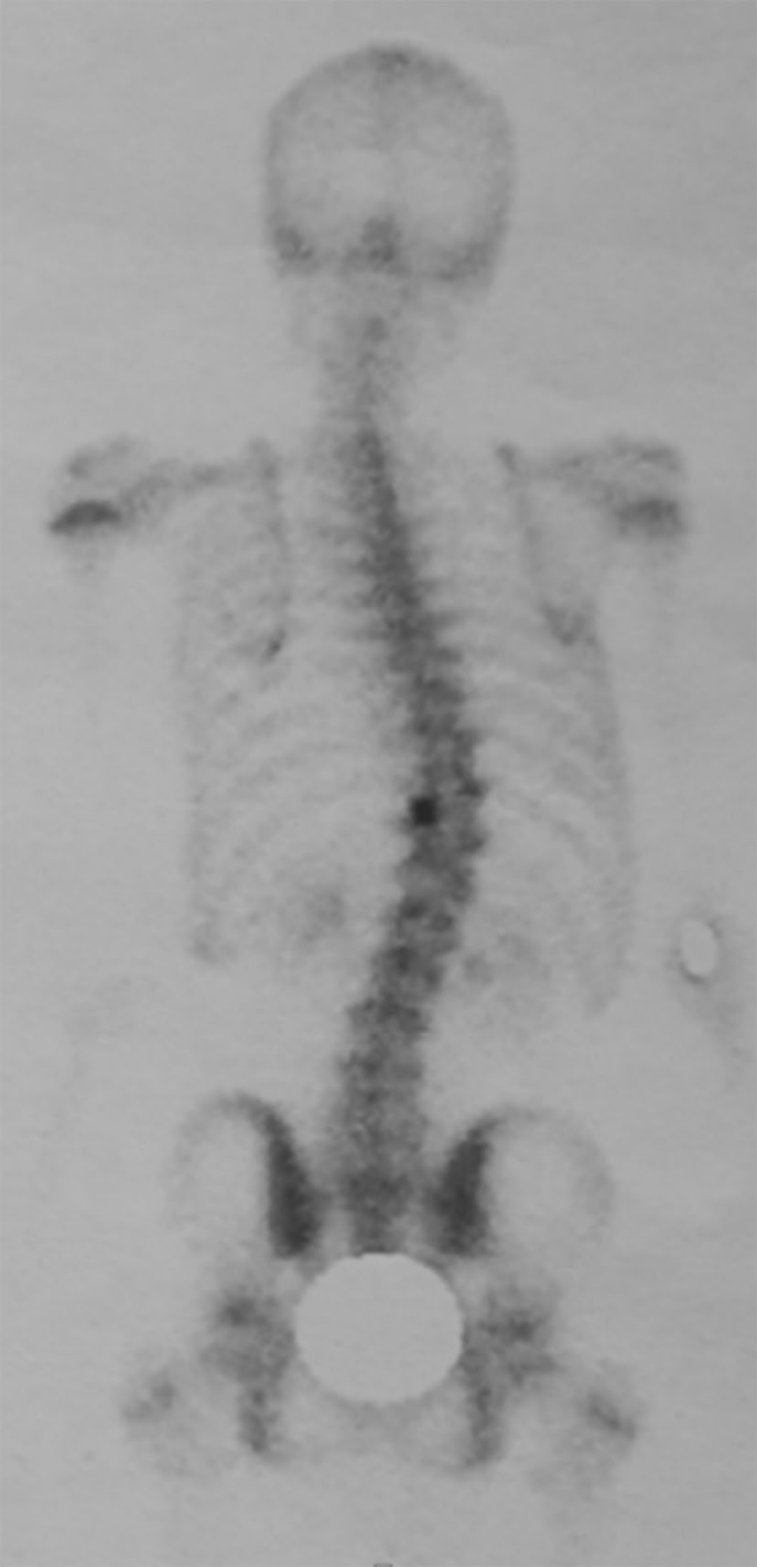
Bone scintigraphy: hyperfixation of the left superior articular process of T11
